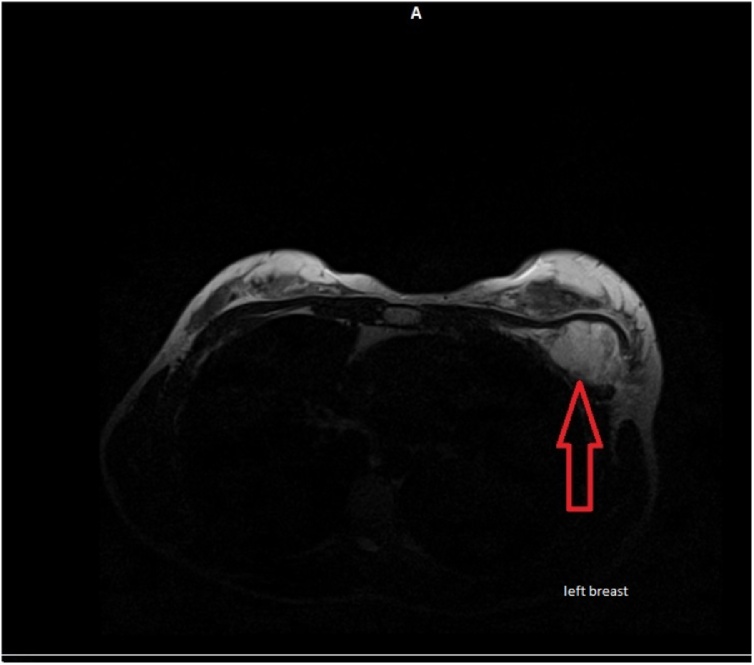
Breast MRI. Arrow pointing towards sub-pectoral Phyllodes tumor.
